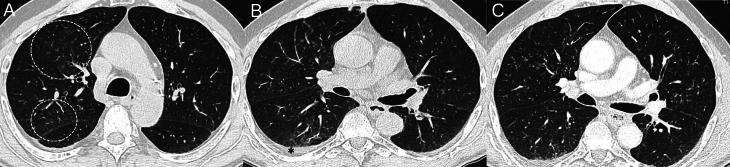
Three different CT scans showing relevant lung involvement. (A) in July 2022, the CT scan showed a diffuse ground-glass area with centrilobular micronodules and bronchiolar wall thickening in the right upper lung lobe (white circles). (B) in December 2022, the CT scan demonstrated the appearance of a new ground-glass area with bronchiolar wall thickening in the right lung with pleural effusion, as indicated by the asterisk (*). 0(C) in February 2024, the last CT scan showed multiple centrilobular micronodules with bronchiolar wall thickening diffuse in both lungs.
